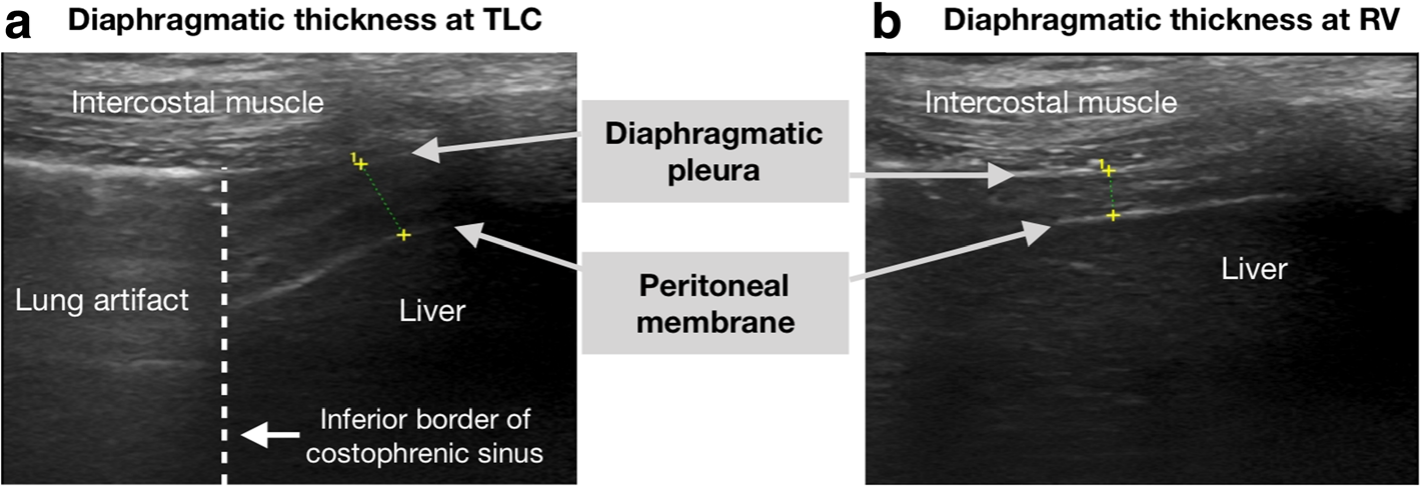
Ultrasound diaphragm shows three layers of diaphragm and diaphragmatic thickness at total lung capacity (TLC) in (a) and residual volume (RV) in (b). The inferior border of the costophrenic sinus can be demonstrated clearly in (a) by the level of lung artifact caused by the ultrasound reflected by the air in the lung
